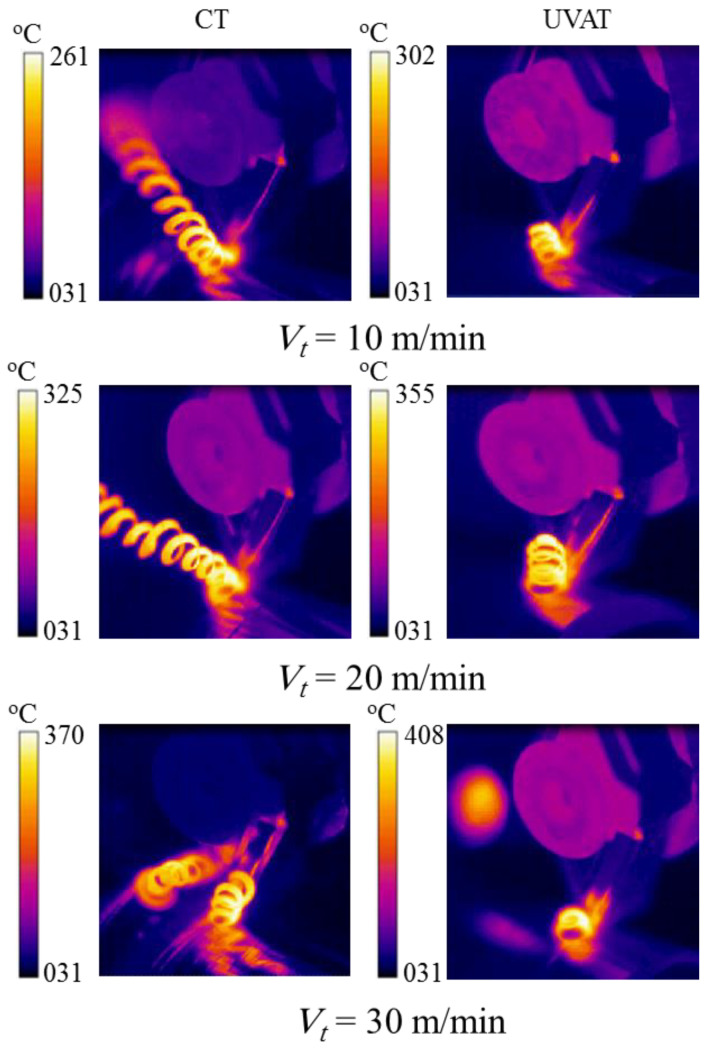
Measured temperature in CT and UVAT.
